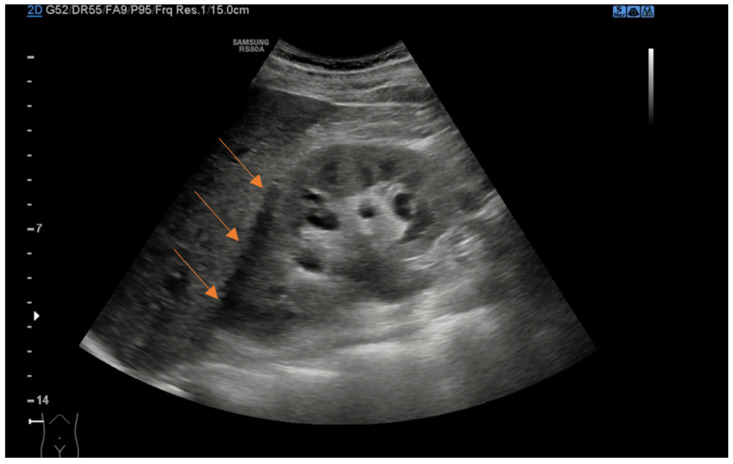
Coronal ultrasound view of right kidney demonstrating anechoic perinephric fluid (arrows).
